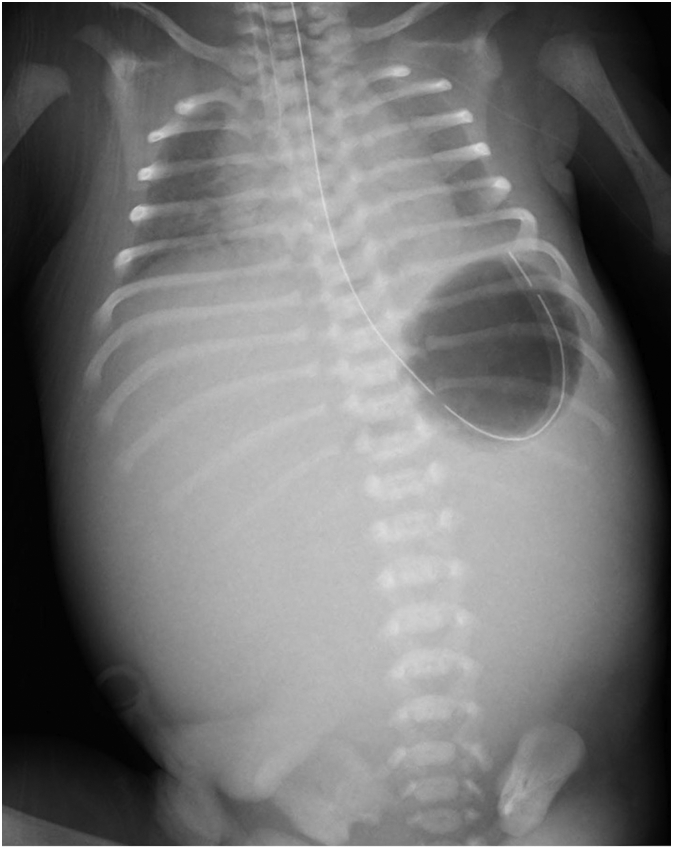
Postnatal abdomen supine X-ray shows stomach gas without bowel gas passage and prominent diaphragmatic elevation.
